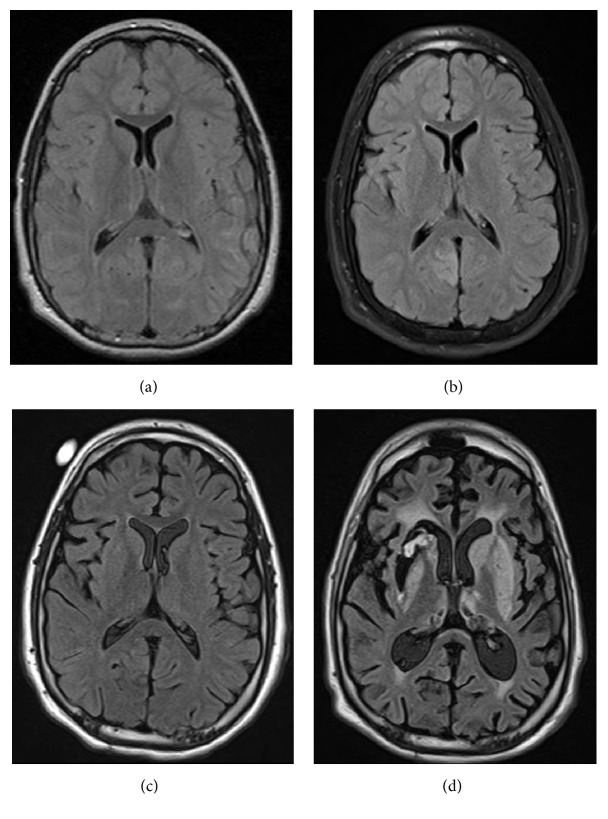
Serial brain magnetic resonance imaging (MRI). Consecutive fluid attenuated inversion recovery sequences (FLAIR) of brain MRIs of the patient with super refractory status epilepticus in a medically induced coma. The serial images show development and progression of diffuse cerebral atrophy.
